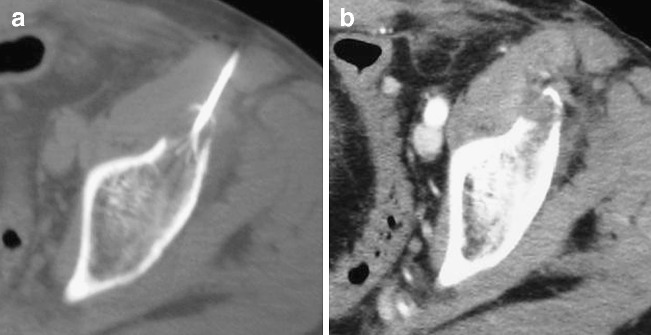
Computed tomography scan a during and b after the radiofrequency session with the patient in a supine position. The electrode is deployed inside the metastatic lesion of the left acetabulum (metastasis from breast cancer). There is no enhancement after intravenous contrast media administration. Before the radiofrequency session, the average pain score was 7. During the first 24 h after radiofrequency, it was 4 and 1, 4 and 8 weeks later the average pain scores were 3, 2 and 0 respectively
